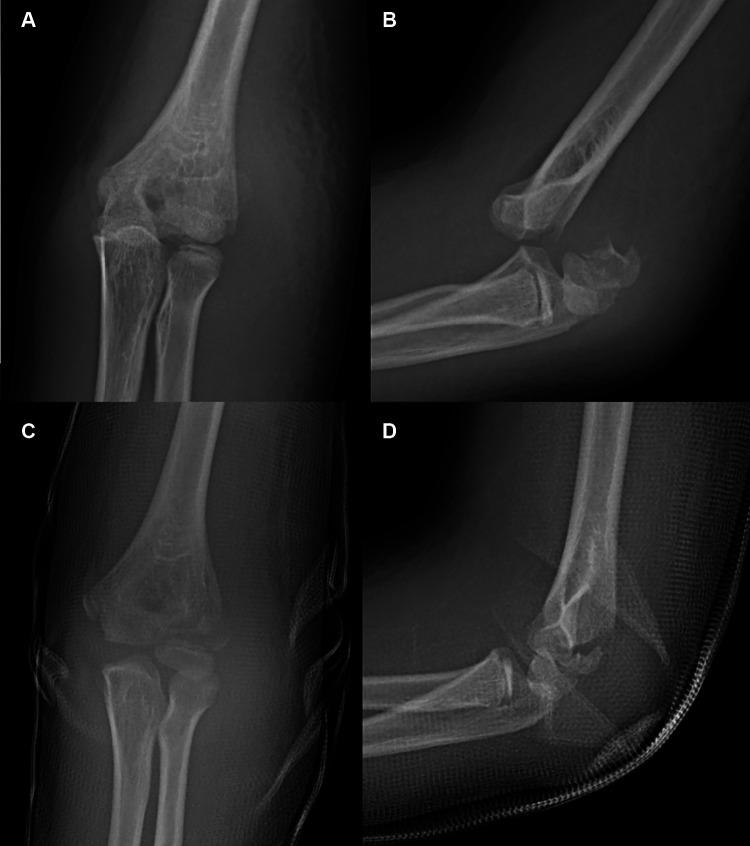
Typical x-ray of Type III fractures. AP (A) radiograph and lateral (B) radiograph showing a fracture with dislocation of the elbow. The fracture is classified as Type III. AP (C) radiograph and lateral (D) radiograph showing a Type III fracture reduced to Type I after reduction of the dislocation.
